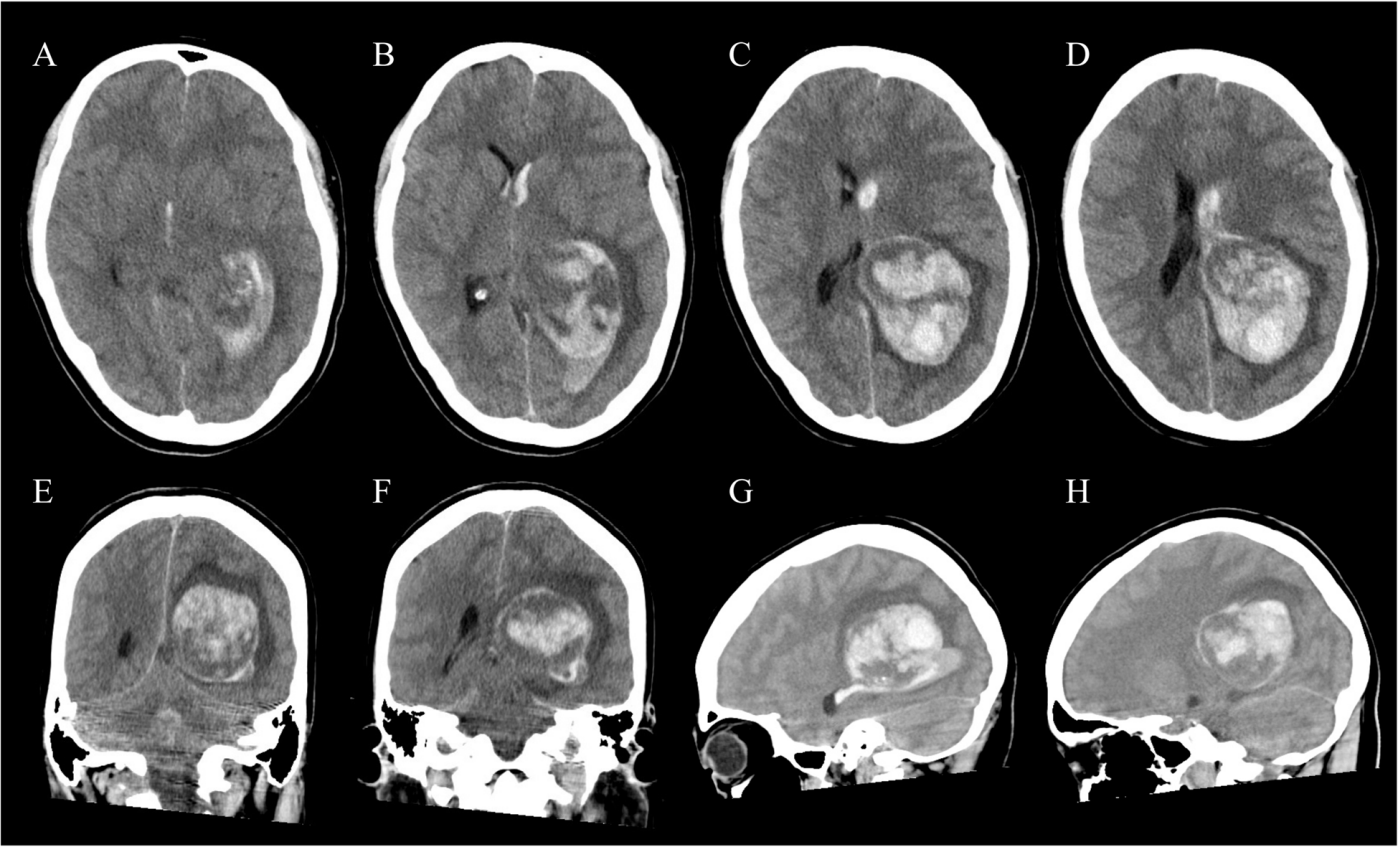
Non-contrast axial (a-d), coronal (e, f), and sagittal (g, h) CT scans on admission revealing a large heterogeneous mass lesion in the trigone of the left lateral ventricle with intraventricular hemorrhage extending to the right lateral ventricle
